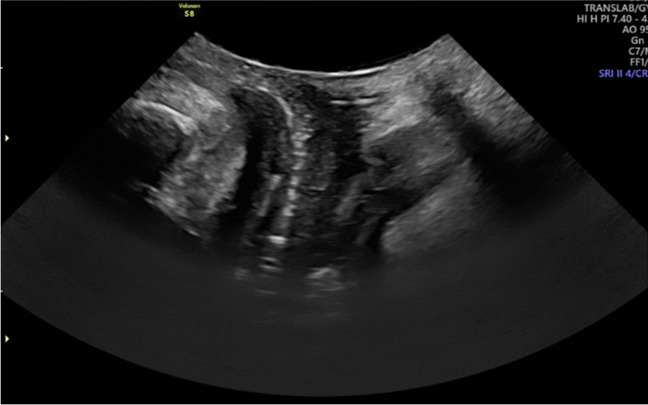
Transperineal ultrasound at rest
